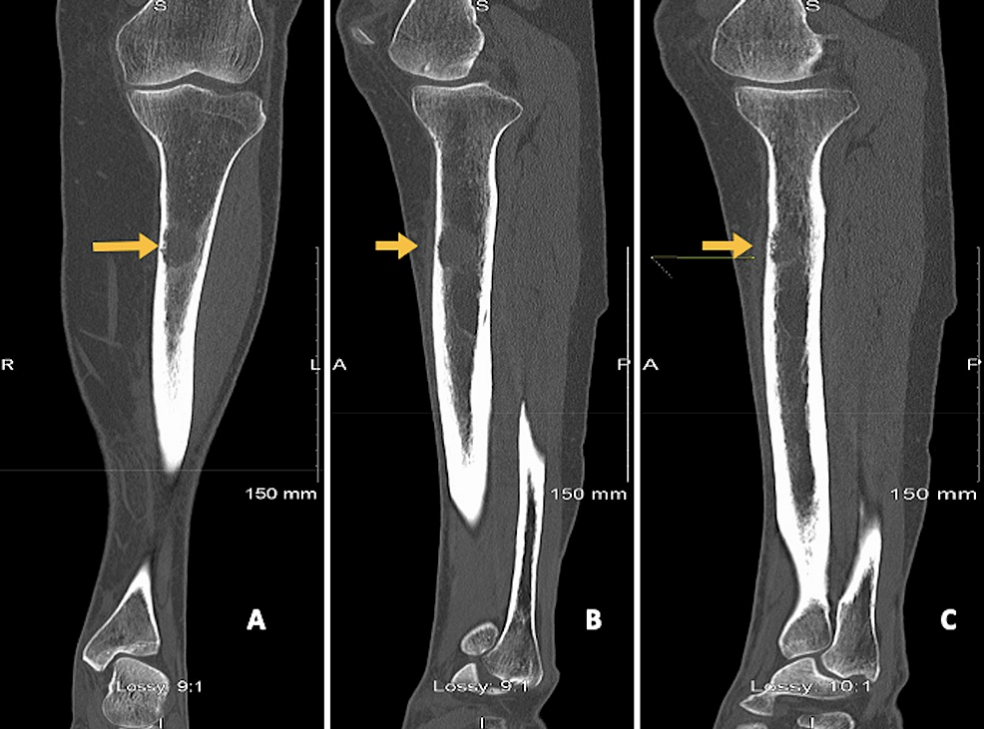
Computed tomography (CT) scan of the left tibia and fibula (A) Coronal non-contrast CT scan view of the left tibia and (B-C) sagittal non-contrast CT scan views of the left tibia showing a well-defined osteolytic tumour of the proximal shaft/diaphysis of the left tibia (arrow) seen with a moth-eaten appearance of the internal table of the cortical anteromedially. Appearances are suggestive of myeloma, metastatic deposit, or chondrosarcoma as the main differentials.
